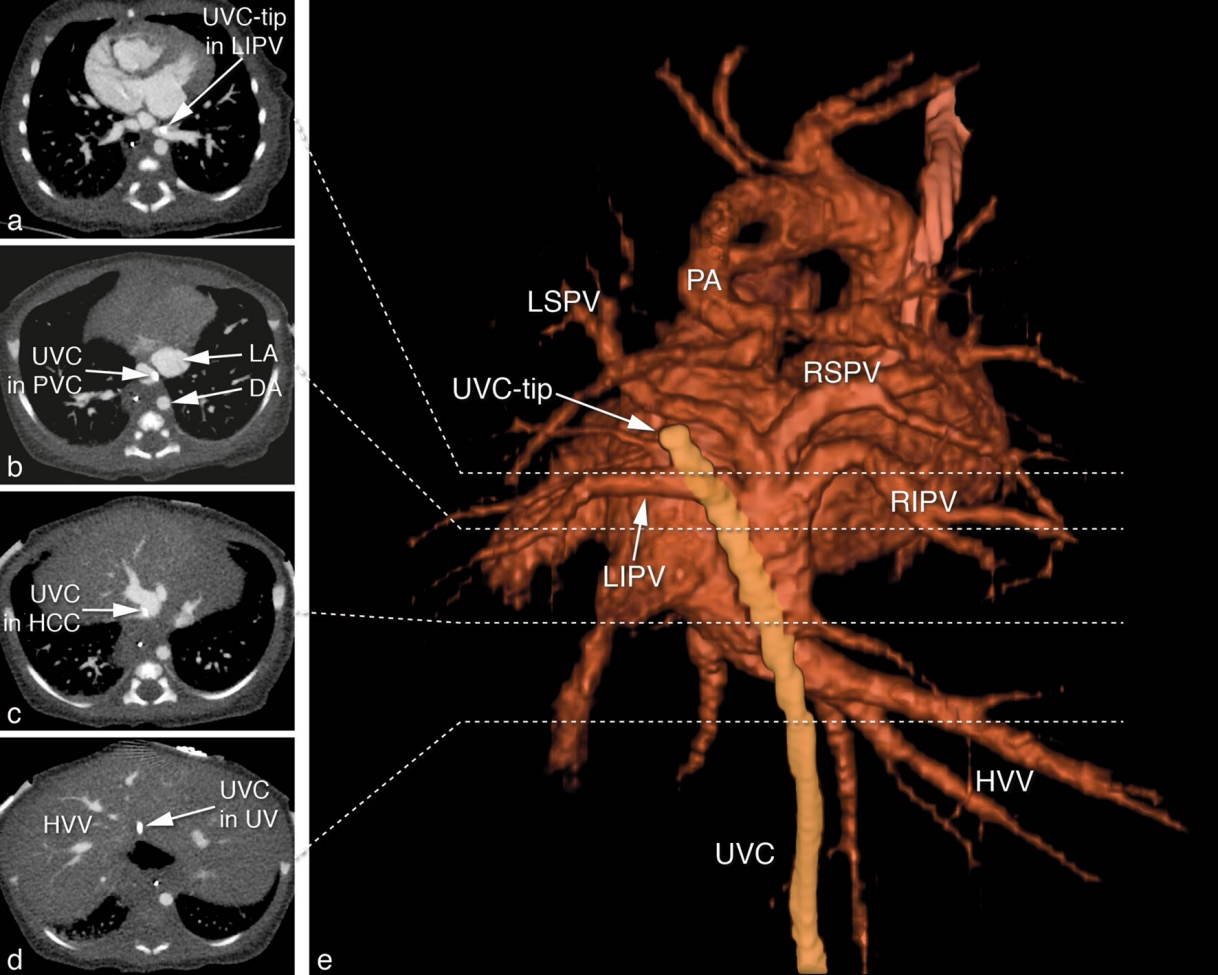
CT images demonstrating the presence of the UVC in a pulmonary vein
